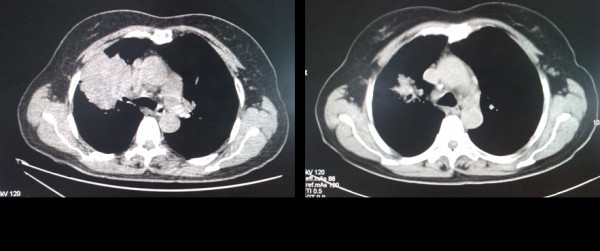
Case 21 showed that the sizes of both the primary tumor and the mediastinal lymph nodes were found to shrink after gefitinib therapy when examined by thorax CT scan.
